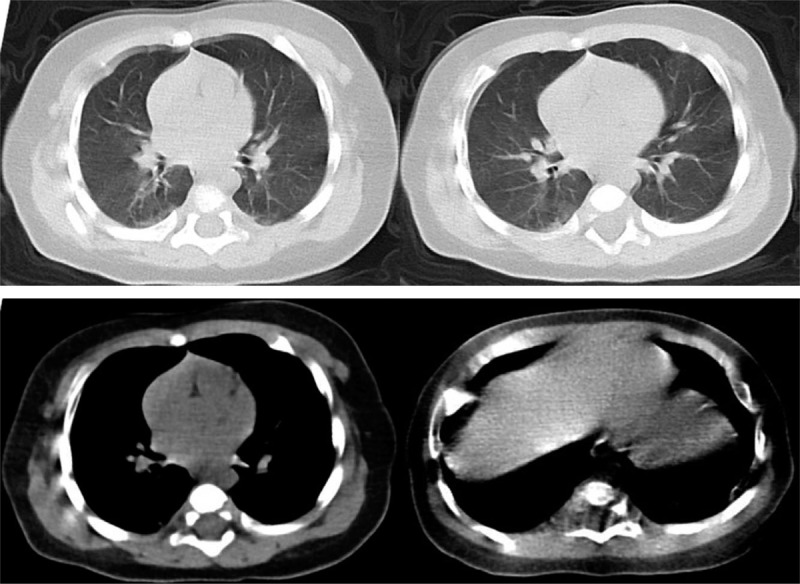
Unenhanced chest CT taken on admission to hospital showing small nodules in the bilateral upper lobe and the dorsal segment of the lower lobe; mainly distributed along the subpleural area; bronchovascular bundles thickening. There is no pleural effusion or lymphadenopathy.
